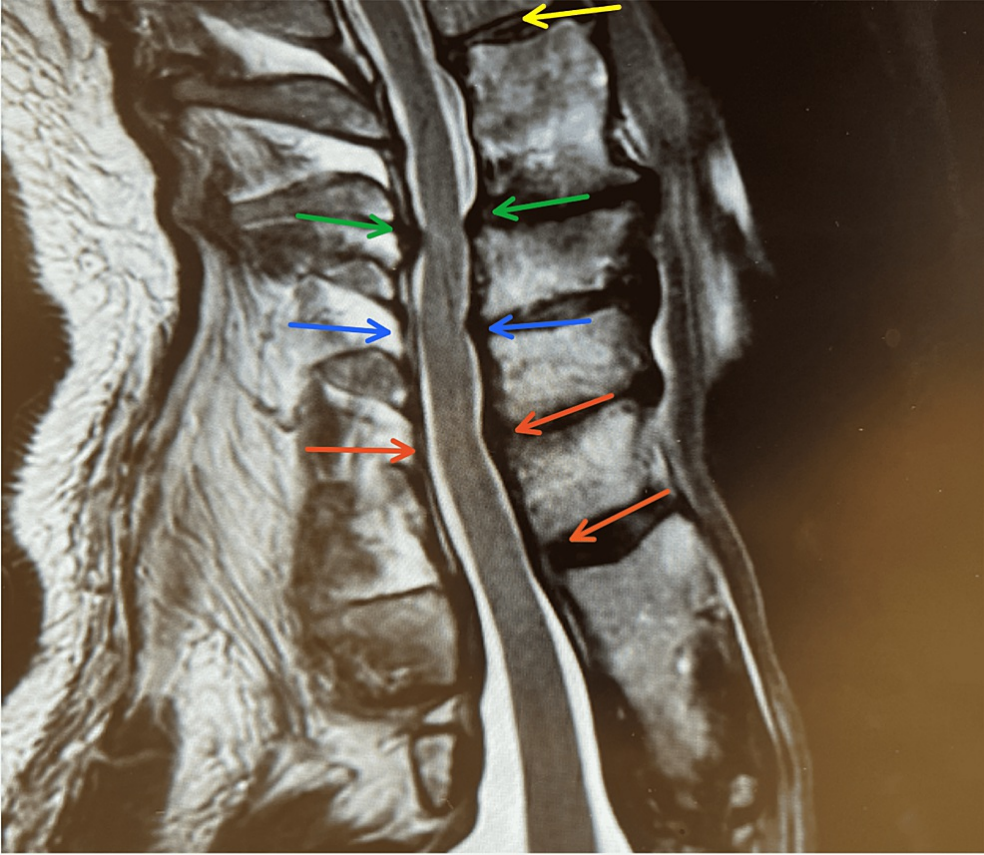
MRI of the cervical spine C2-C3: Small central disc protrusion and bilateral facet arthrosis causing mild central canal stenosis without neuroforaminal narrowing (yellow arrow). C3-C4: Bilateral facet arthrosis and uncovertebral joint osteophytes causing mild to moderate bilateral neuroforaminal narrowing without central canal stenosis. Bilateral foraminal nerve root encroachment (green arrows). C4-C5: Central disc protrusion causing moderate central canal stenosis. There were bilateral uncovertebral joint osteophytes and facet arthritis causing severe right and moderate left neuroforaminal narrowing with right foraminal C5 nerve root impingement and left foraminal C5 nerve root encroachment (blue arrows). C5-C6: Posterior disc osteophyte complex causing moderate to severe central canal stenosis. There are bilateral uncovertebral joint osteophytes and facet arthrosis resulting in severe bilateral neuroforaminal narrowing with bilateral foraminal nerve root impingement (red arrows). C6-C7: No central canal stenosis or neuroforaminal narrowing (orange arrow).
